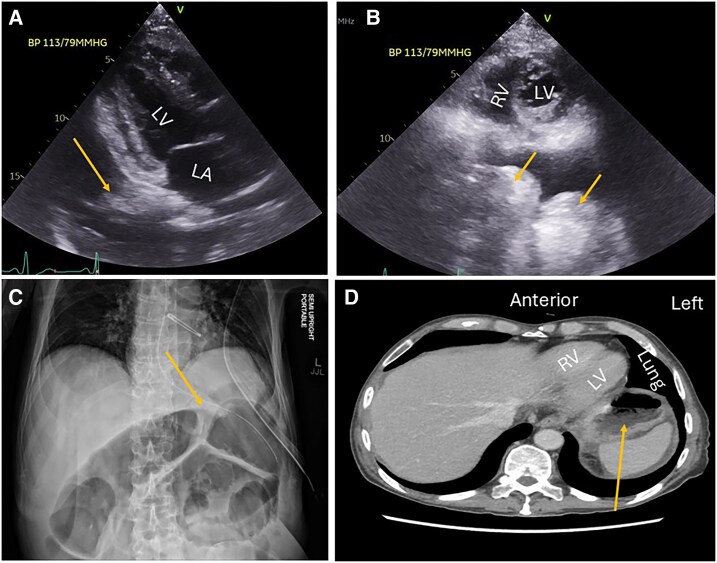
Apical three-chamber view (*A*) showing a bright structure (arrow) related to the posterior wall of the left atrium. Parasternal short-axis view (*B*) showing two large bright white space occupying structures (arrows) related to the posterior wall of the left ventricle at the mid-cavitary level. X-ray (*C*) showing the lower chest and upper abdomen with severely distended bowel. Transverse computed tomography scan (*D*) of the upper abdomen showing distended bowel loops (arrow). LV, left ventricle; LA, left atrium; RV, right ventricle.
